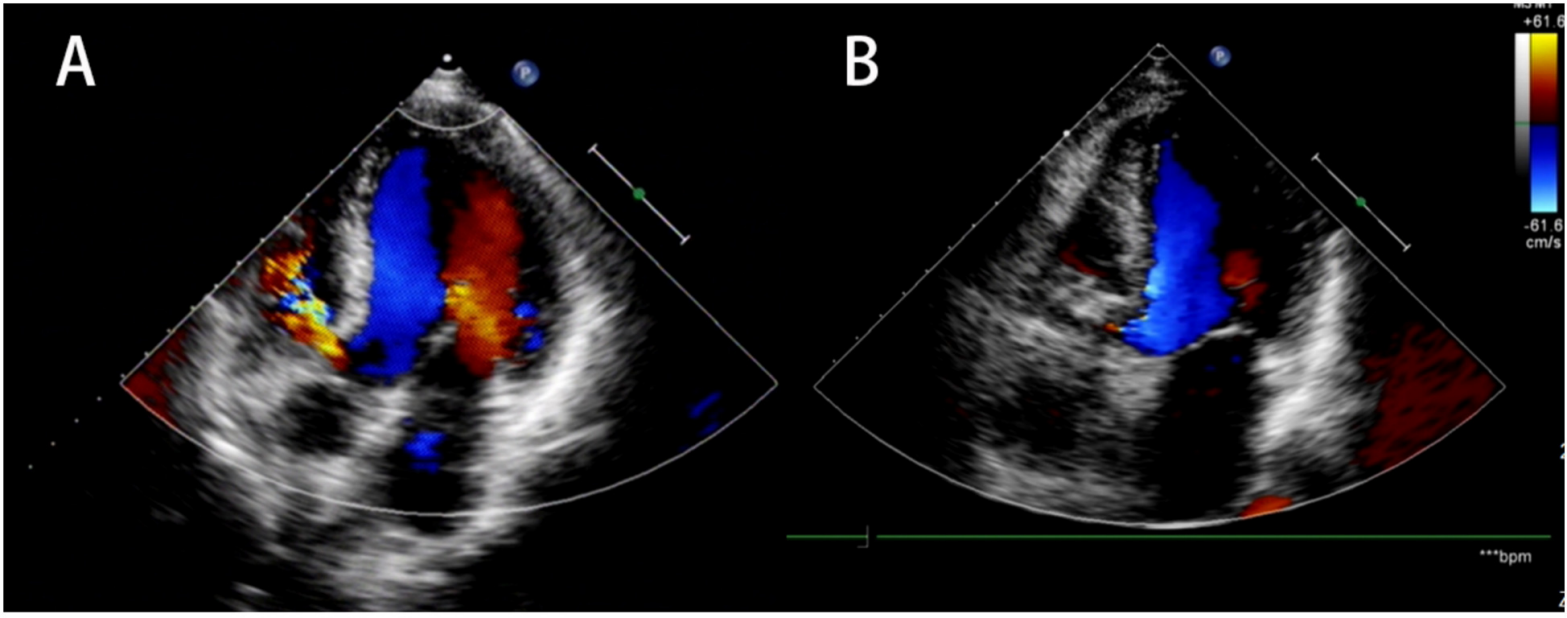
Preoperative and postoperative TTE. (A) Preoperative ultrasound evaluation. (B) Postoperative TTE reexamination showed optimal placement of the occluder, no residual shunt, and no new onset of aortic or tricuspid regurgitation. TTE, transthoracic echocardiography.
